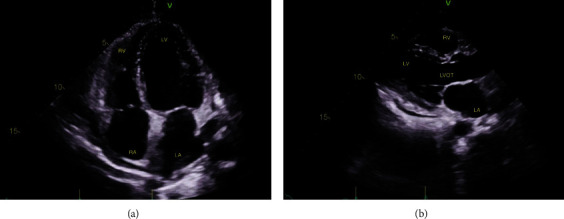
Transthoracic echocardiogram 4 weeks since admission. The left ventricle has normal size. LVEF is 55-60%. LV strain is -19%. The right ventricle free wall is thick, and mitral valve leaflets are thickened. Small-to-moderate circumferential pericardial effusion. There is no evidence of tamponade. (a) Apical 4-chamber view and (b) parasternal long-axis view.
